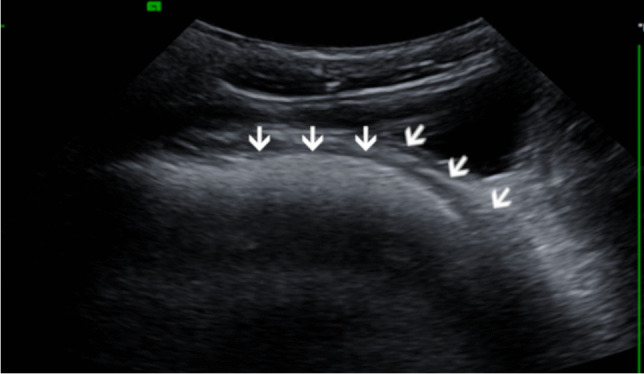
A transverse ultrasound scan of the pelvis in a 10-year-old boy with a clinical diagnosis of constipation shows a huge coprolite (*arrows*)
